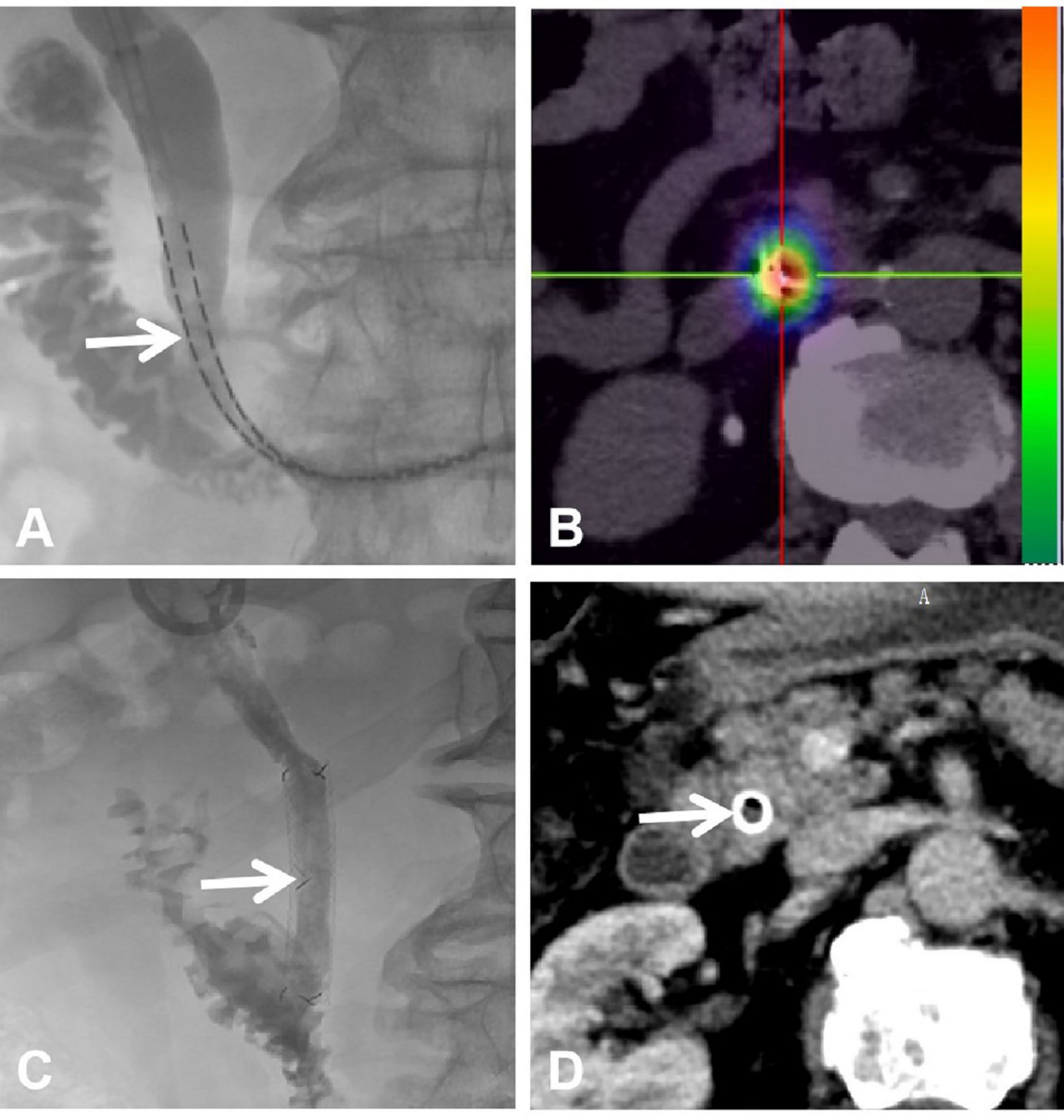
Placement of the brachytherapy drainage catheter and stent patency after 9 months. (A) The brachytherapy drainage catheter was inserted across the PC to the duodenum. (B) SPECT/CT scan showing radiation emitted by ^125^I brachytherapy within the PC. (C) Biliary stent after brachytherapy showing stent patency. (D) CT scan showing stent patency at the 9-month follow-up.
